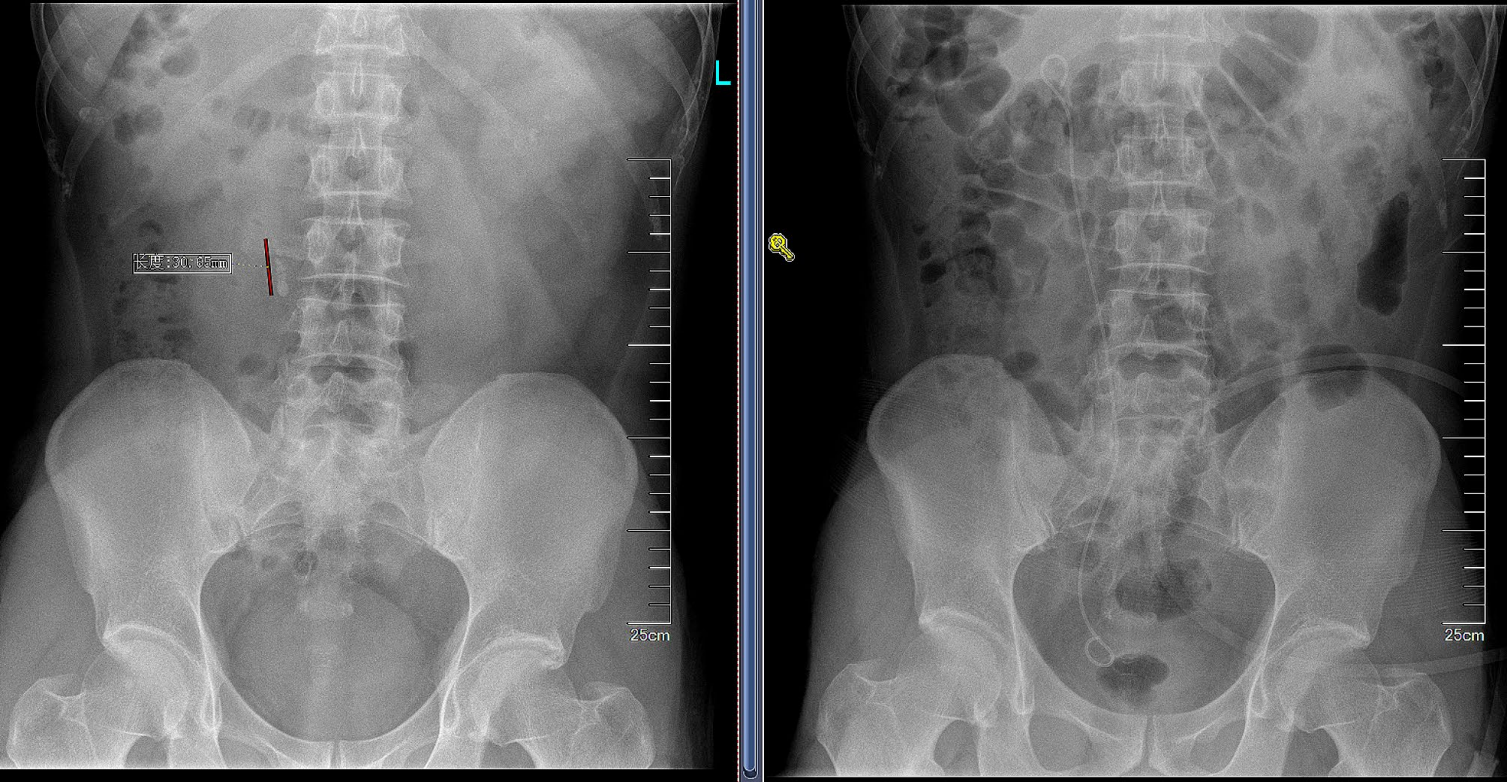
Preoperative and postoperative kidney, ureter, and bladder radiograph (KUB)
